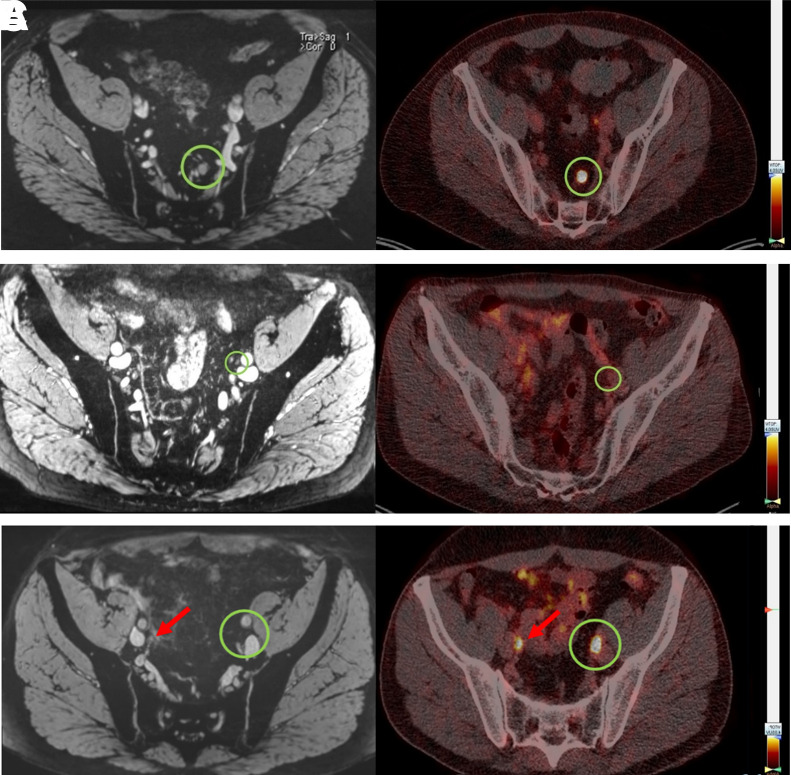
Examples of iron-sensitive T2*-weighted fat-saturated nano-MRI images (left) and PSMA PET/CT images (right). (A) Seven-millimeter-diameter LN in left pararectal region that is positive on both nano-MRI and PSMA PET/CT. (B) Four-millimeter-diameter LN in region of left external iliac artery that is positive on nano-MRI but negative on PSMA PET/CT. (C) Suspicious LN dorsal to left external iliac artery that is negative on nano-MRI (nano-MRI also shows no left ureter in this area) but positive on PSMA PET/CT. LNs are encircled; arrows indicate right ureter.
